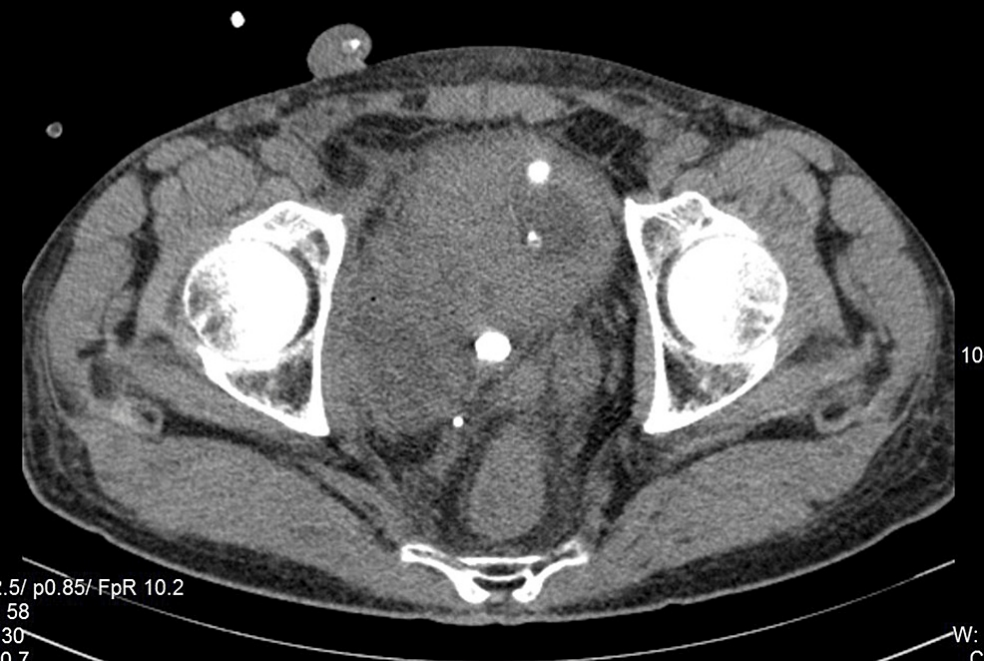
Axial plane of the contrast CT scan showing both extravesical and vesicle calculi. CT, computed tomography
